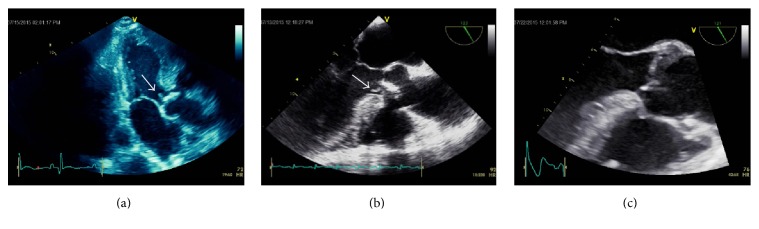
(a) Transthoracic echocardiogram image demonstrating a vegetation (arrow) on the ventricular surface of the aortic valve. (b) Transesophageal echocardiogram image demonstrating the small vegetation on the ventricular surface of the aortic valve, as well as aortic valve thickening in patient with known bicuspid aortic valve. (c) Follow-up transesophageal echocardiogram demonstrating no evidence of previously visualized vegetation, suggesting its resolution or embolism. No evidence of pseudoaneurysm was detected on serial echocardiographic studies.
